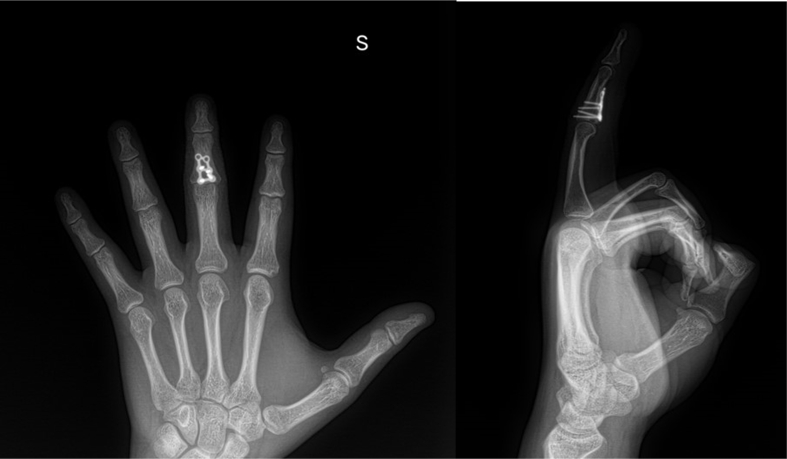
Post-operative X-ray with surgical hardware in place. A post-operative lateral X-ray of the left hand demonstrating successful placement of the adapted Medartis TriLock plate at the PIP joint of the third digit. The image shows the alignment and stabilization achieved through the surgical procedure, indicating the beginning of the patient's journey to recovery.
